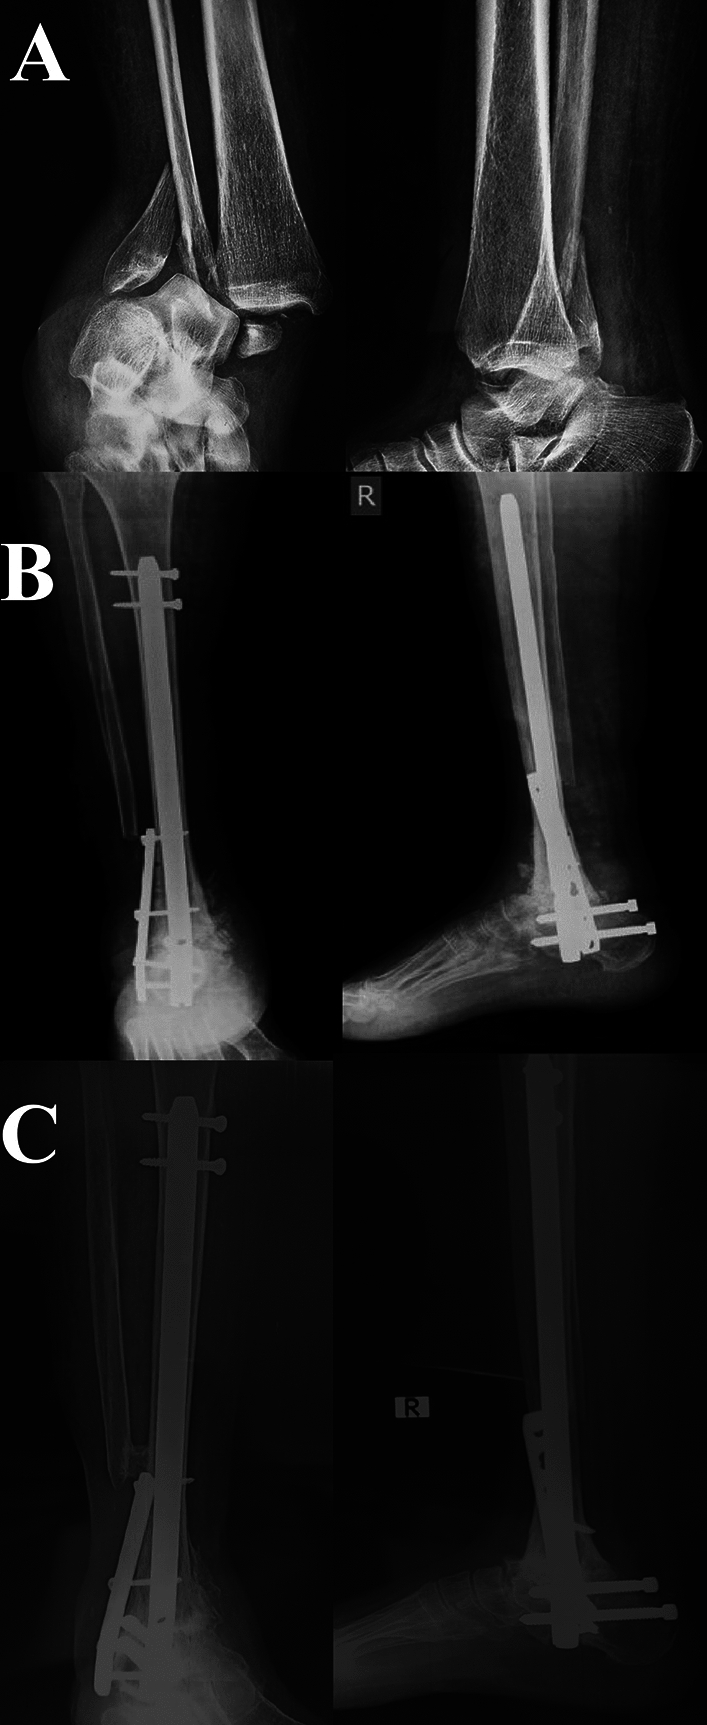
A female patient 63 years old, presented with ankle fracture dislocation (OTA/AO 44B3) (HbA1C: 7.7 and AFDA score 8). A preoperative radiographs. B Immediate postoperative radiographs. C At 12 months, follow-up radiographs showed stable implants and ankle arthrodesis
